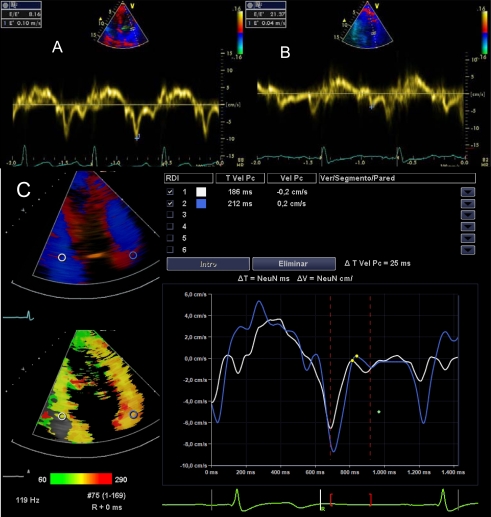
E/E´ratio. (A) Normal, (B) elevated, (C) E/E´ estimation with advanced echocardiographic software.
